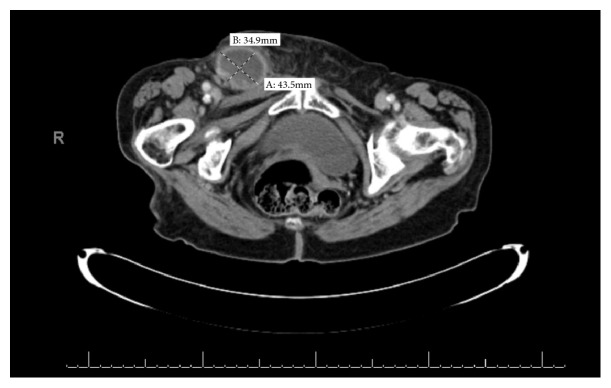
CT abdomen/pelvis image of right sided De Garengeot's hernia with adjacent abscess measuring 4.3 cm x 3.5 cm transversely.
